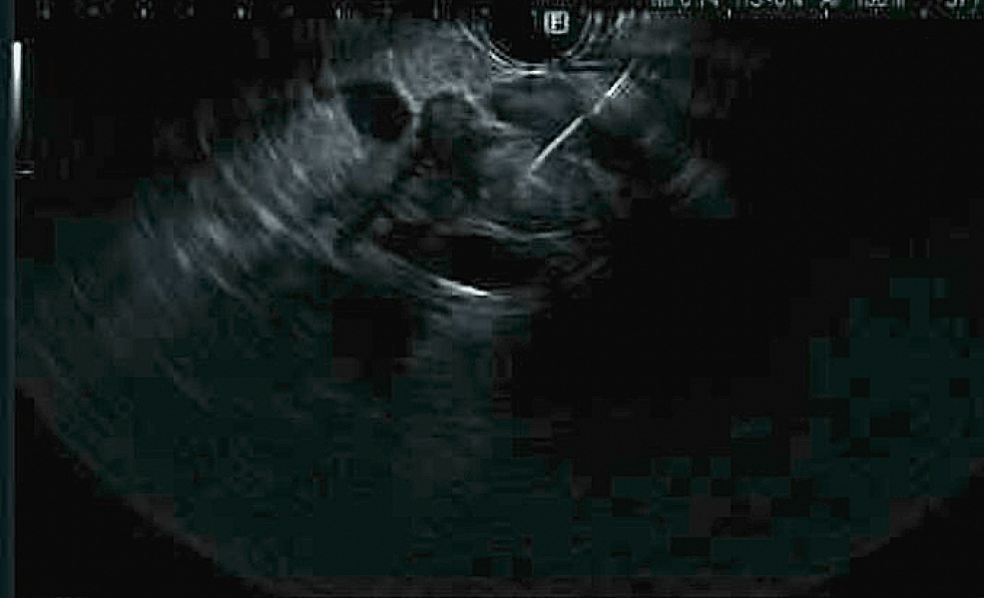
Endoscopic ultrasonography (EUS) imaging with fine needle aspiration (FNA) of the pancreas
